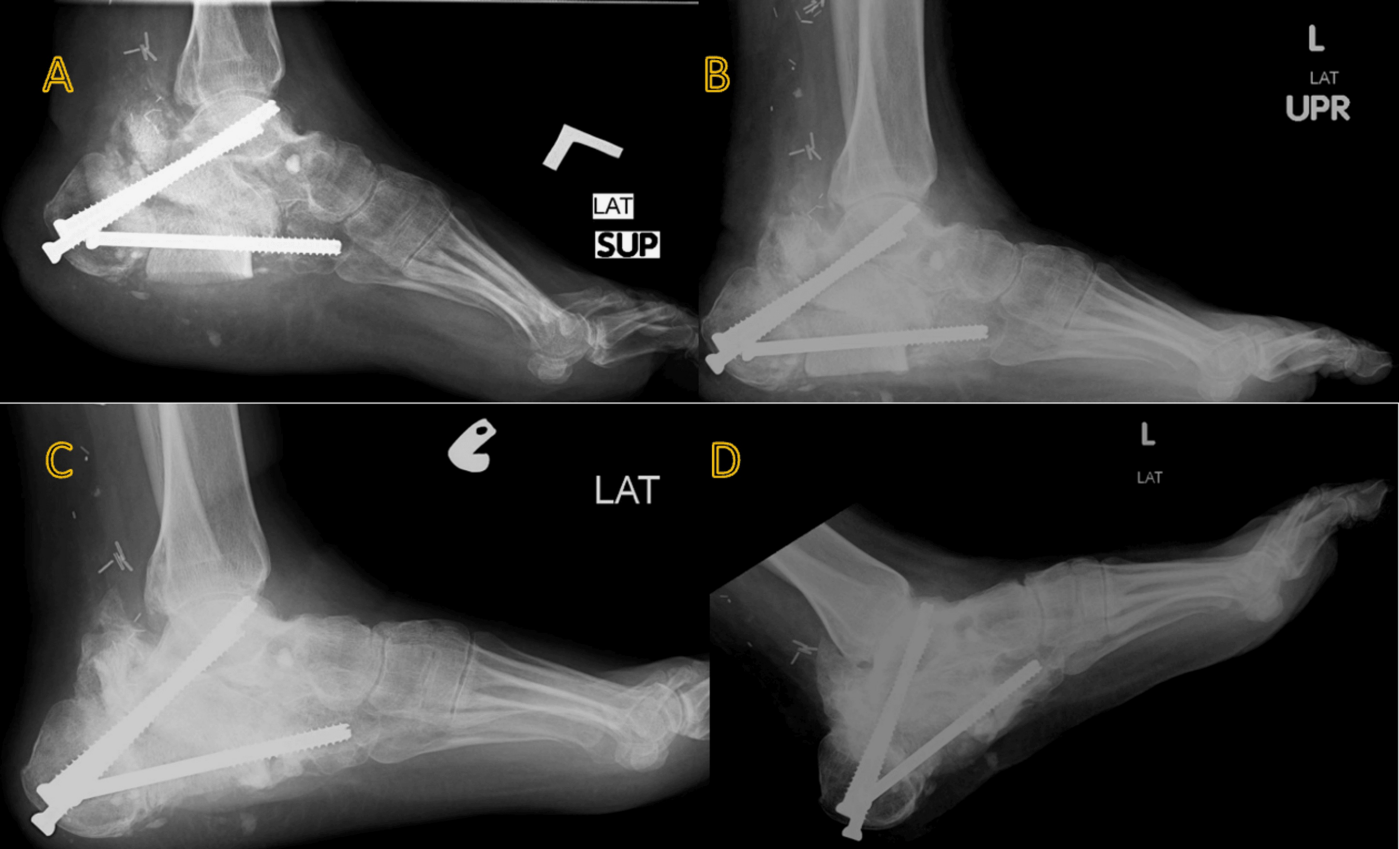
Left lateral foot X-ray (A) Two months post-procedure; (B) Six months post-procedure; (C) One year post-procedure; (D) Eighteen months post-procedure
